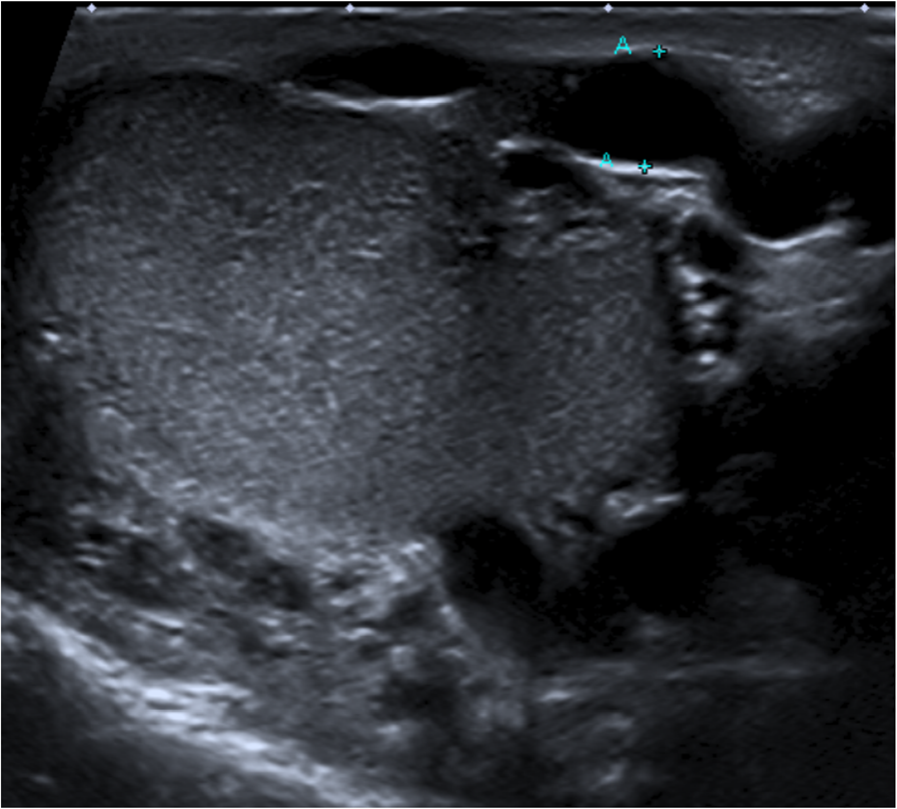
Ultrasound of the left testis, 5 months prior to the ER visit, during a Valsalva Manoeuvre. A clear varicocele of 4.3 mm is visible (A-A)
